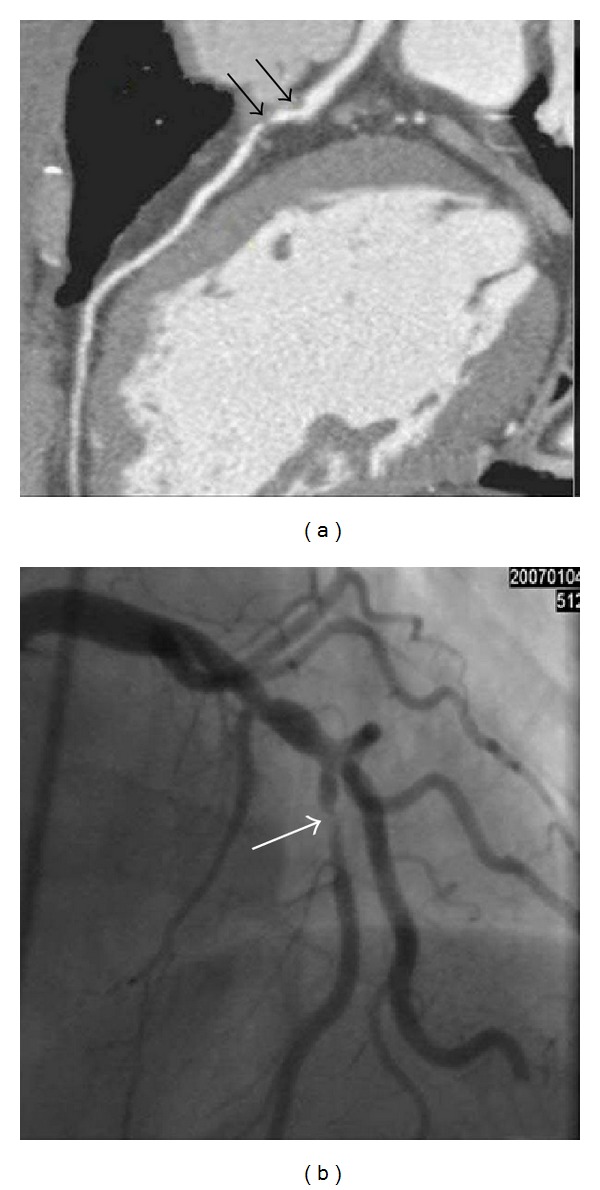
A 66-year-old male with zero calcium score. (a) A computed tomographic angiogram shows soft plaques (black arrows) at the left anterior descending artery (LAD) with severe stenosis. (b) Conventional coronary arteriogram confirms the severe stenosis of the LAD (white arrow).
